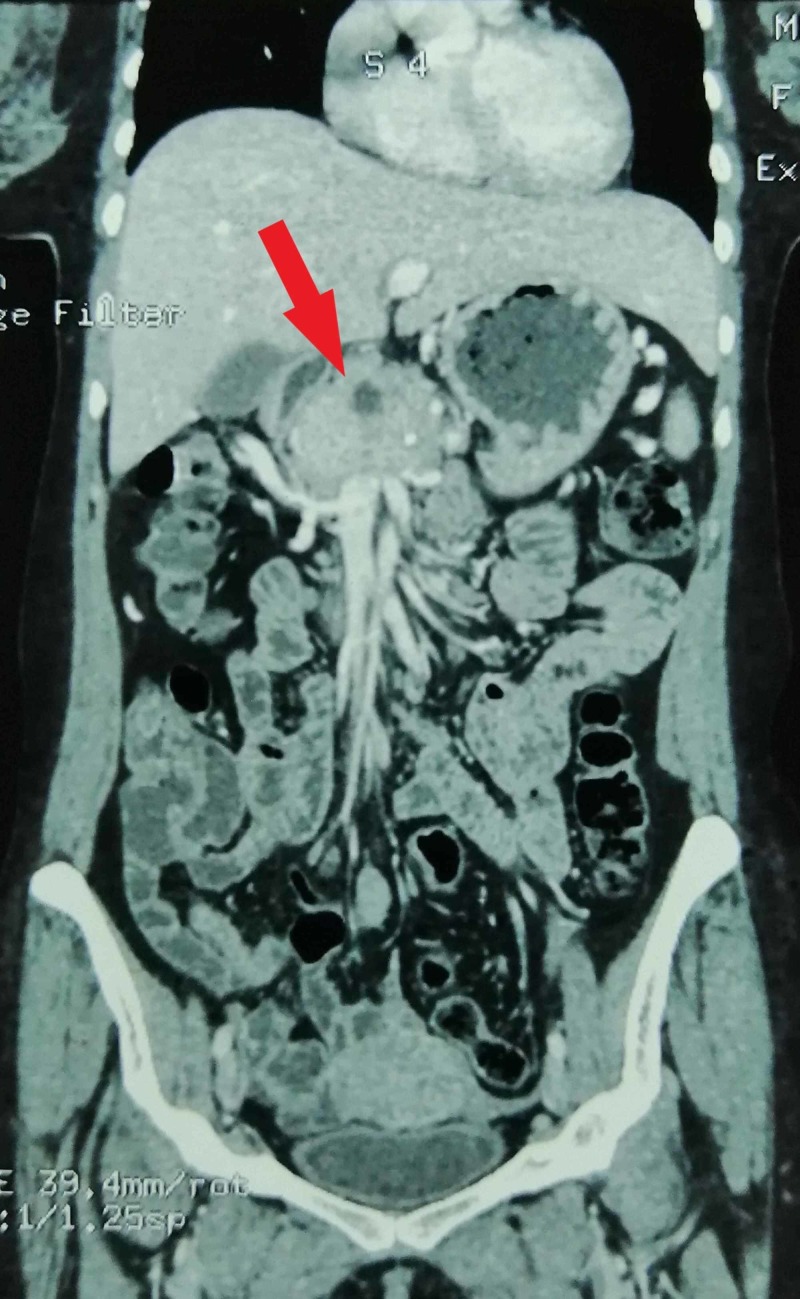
Coronal view of abdominal CECT showing a hyperdense, ill-defined mass arising from the pancreatic body CECT, contrast-enhanced computed tomography
